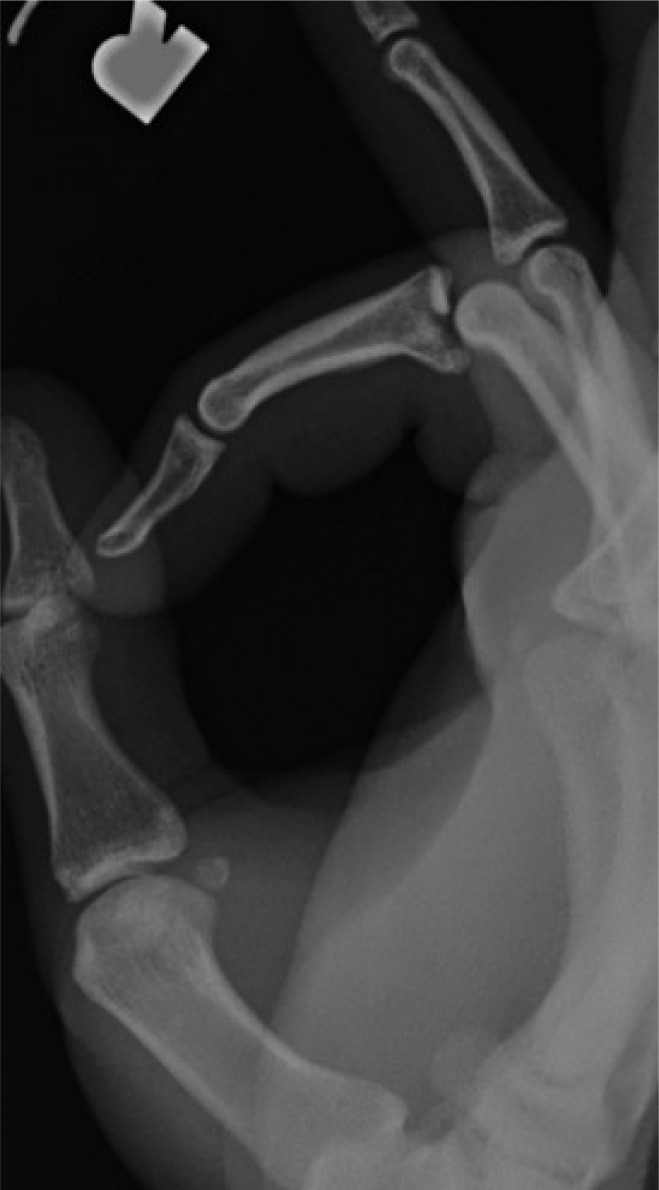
Patient 5 preoperative radiograph.
